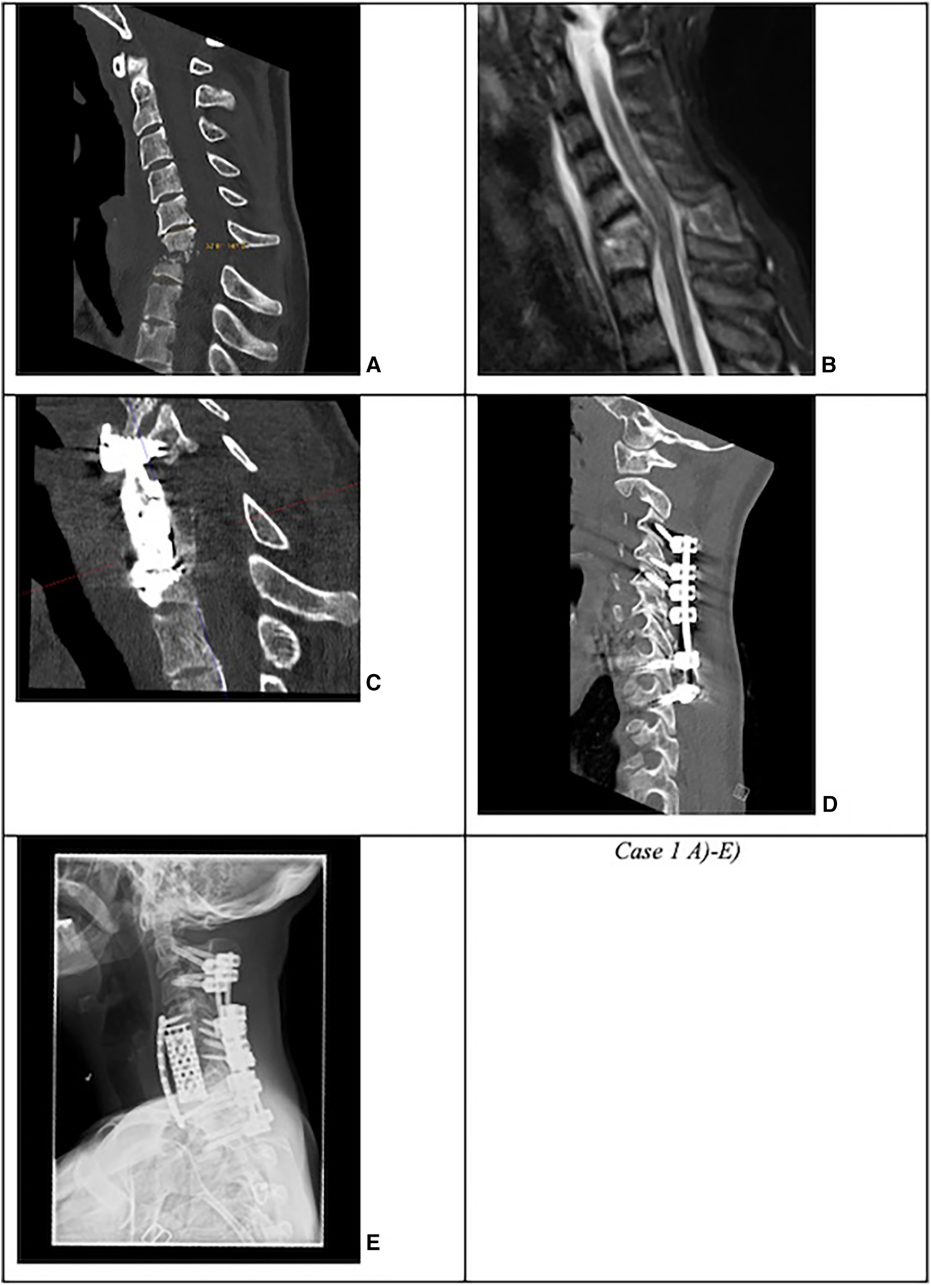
Case 1: Complicated cervical spondylodiscitis in C6/7 with long-segment (three levels) posterior epidural abscess, kyphotic sagittal deformity with sagittal angle of 33°, and progressive osteomyelitis in a drug abuser. (A) CT and (B) MRI with C6/7 spondylodiscitis with 33° kyphotic deformity. (C) First revision surgery due to cage subsidence with dorsal spondylodesis extension to C4 and anterior plating. (D) Secondary lateral mass screw loosening in C3 and C4. (E) Second revision surgery with pedicle screw placement in C2 and C3.
